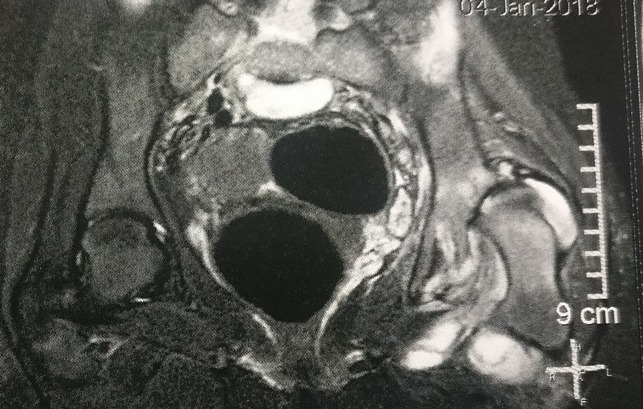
Radiograph demonstrating the coronal T2 fat saturation dysplastic head with adjacent hyperintensity in the soft tissues and localized fluid collection.
